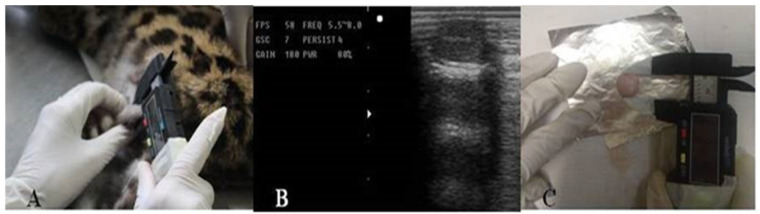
Methods for testicular measurements used to calculate testicular volume in adult felines. (A) Method 1: using a digital pachymeter before orchidectomy. (B) Method 2: ultrasound measurement. (C) Method 3: using a digital pachymeter after orchidectomy.
